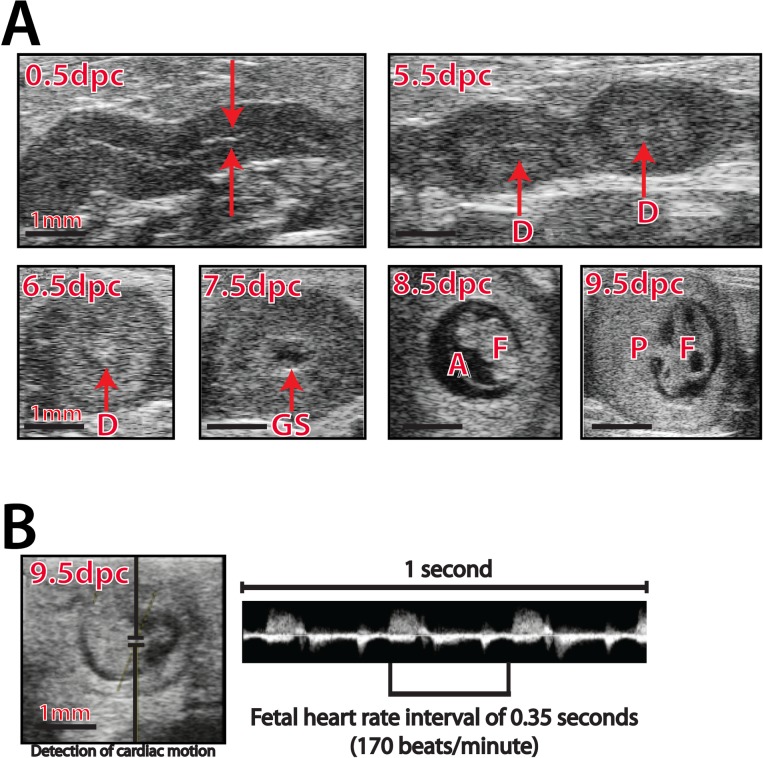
High Frequency Ultrasound detection of implantation site development and monitoring of fetal growth throughout gestation. (A) The morning after overnight mating was considered 0.5 d.p.c. The endometrial lining, developing implantation sites and fetal structures were visualized from 0.5 d.p.c. to 9.5 d.p.c. Implantation site number and location, decidualized endometrium (D) on 6.5 d.p.c, gestational sac (GS) on 7.5 d.p.c, fetus (F), amniotic membrane (A) on 8.5 d.p.c, and placenta (P) and fetus (F) on 9.5 d.p.c. are indicated by red arrows. (B) At 9.5 dpc, pulse wave Doppler placed over the cardiac ventricles allows for recording of fetal viability as measured by cardiac activity and heart rate; a typical pulse waveform pattern is shown.
